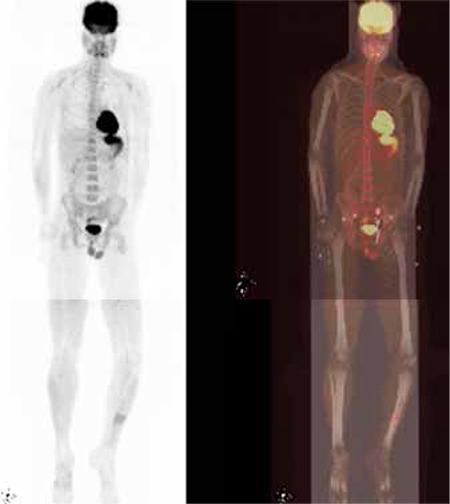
MIP (left) and PET/CT fusion (right) images of repeated FDG PET/CT scan after systemic chemotherapy and external local radiotherapy to theleft tibia. The uptake in bilateral enlarged kidneys, shown in previous PET/CTscan, was completely regressed whereas very intense accumulation at theleft tibia showed near complete regression. Additionally, there was slightlyincreased heterogenous FDG uptake in the fracture line at the middle partof left tibial diaphysis consistent with inflammatory process.
